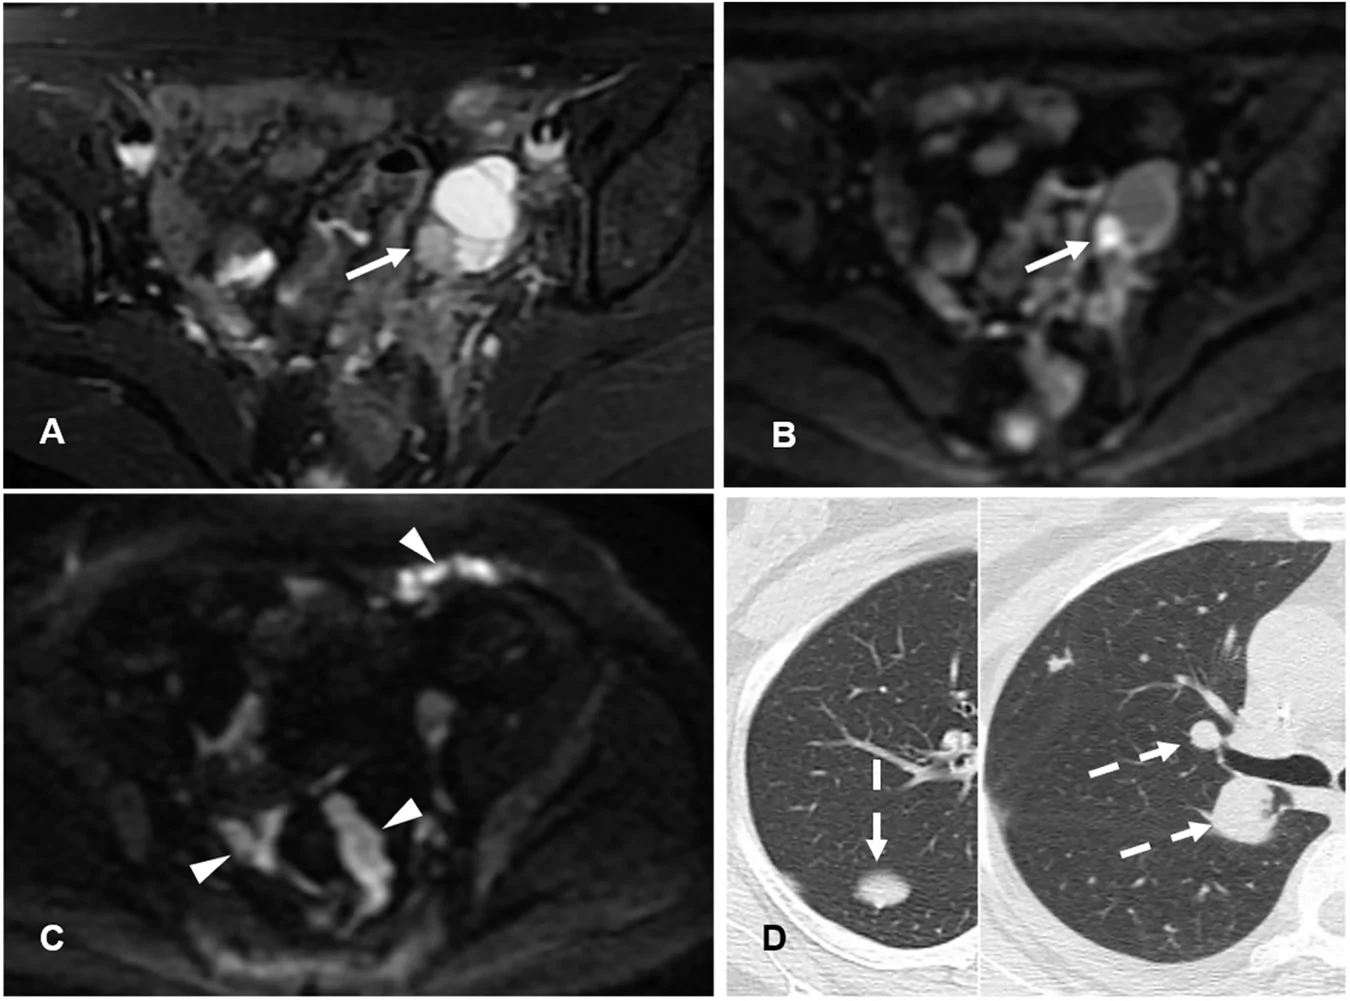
LG-ESS from pelvic MRI and chest CT of a 35-year-old (A, B) woman and a 46-year-old (C, D) woman. Axial T2W (A) and DW (B) images showing an absent uterus with a hyperintense nodular recurrent lesion on the left ovarian surface (arrows). Axial DW (C) image demonstrating multiple hyperintense metastatic lesions in the mesentery and anterior abdominal wall (arrowheads). Chest CT image (D) revealing well-defined nodules pathologically confirmed as metastases (dotted arrows)
